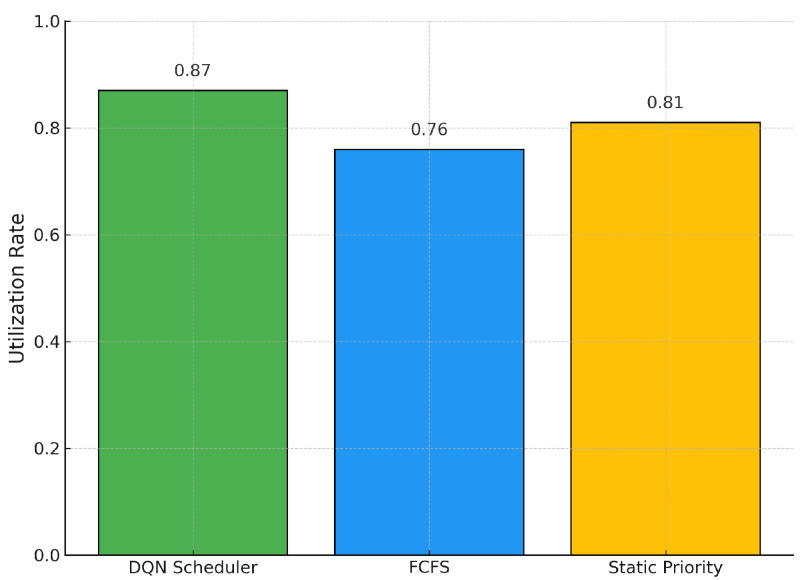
MRI Machine Utilization Rates across Scheduling Policies.
